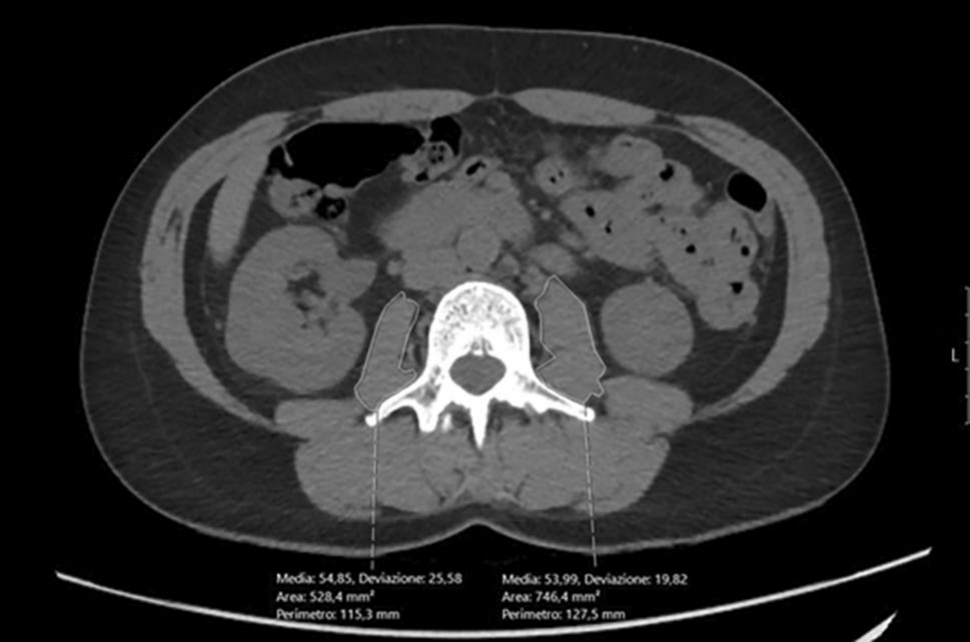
Computerized tomography (CT) abdomen image of a 49-year-old patient showing standardized measurements of the psoas area (mm2) and density (HU)
